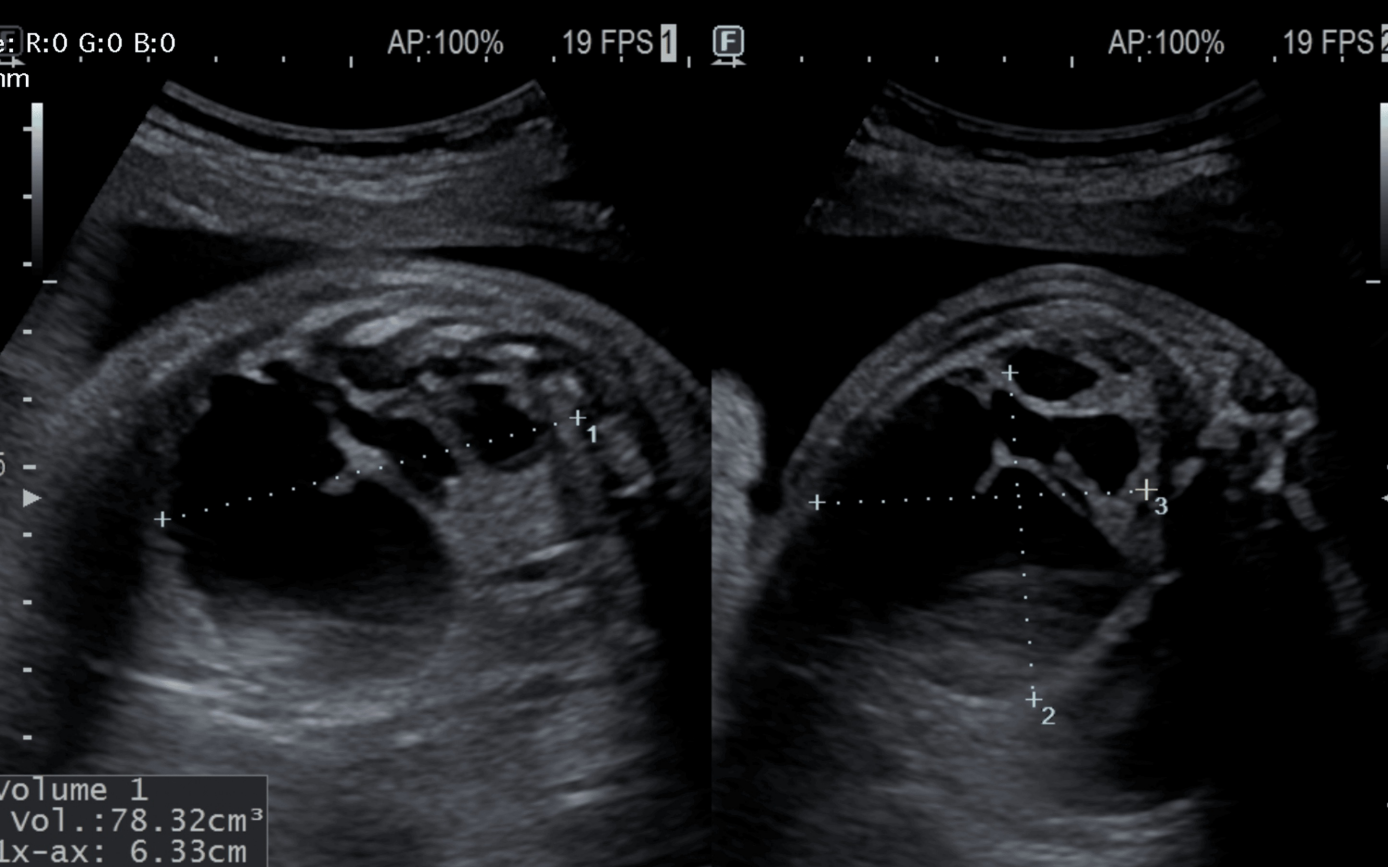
Prenatal ultrasound image demonstrating CPAM volume calculation, obtained by measuring the maximum length, width, and height of the lesion and applying the ellipsoid formula. CPAM: congenital pulmonary airway malformation.
